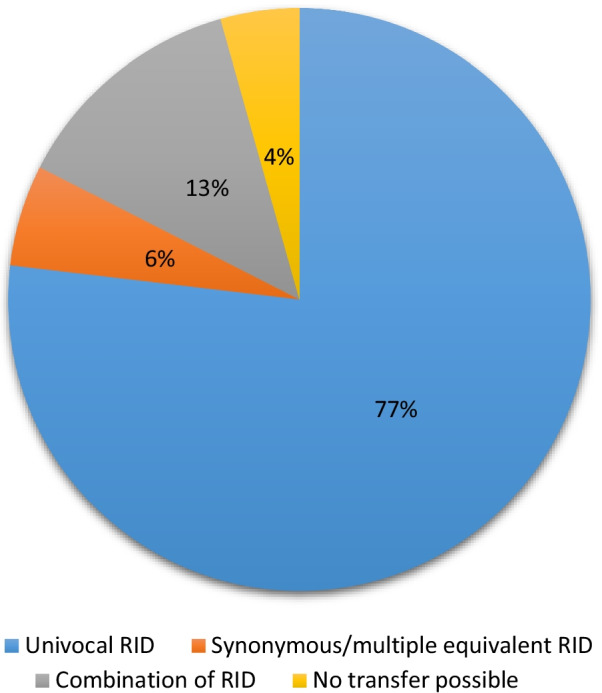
Radlex coverage of terms used in glioblastoma MRI reporting. This pie chart visualizes the broad coverage of elements used in clinical MRI reporting of glioblastomas by the RadLex terminology. RID = RadLex identification number
